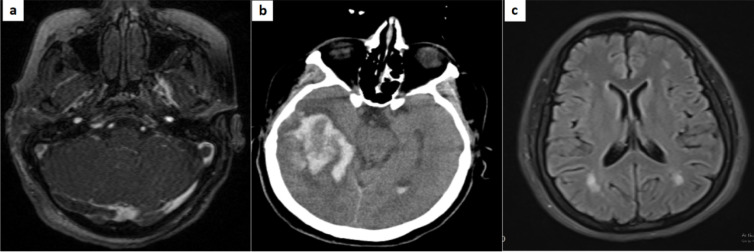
(a, b) Large hyper dense heterogeneous lesion in right temporal lobe with peripheral edema, more evaluated with brain magnetic resonance imaging/venography (MRI/MRV), which showed abnormal signal in right sigmoid sinus compatible with cerebral venous thrombosis; (c) T2 Flair images in a 39-year-old female with COVID-19 shows some hyper intense predominantly subcortical and deep white matter lesions without periventricular and corpus callosum involvement suggestive of acute disseminated encephalomyelitis (ADEM).
